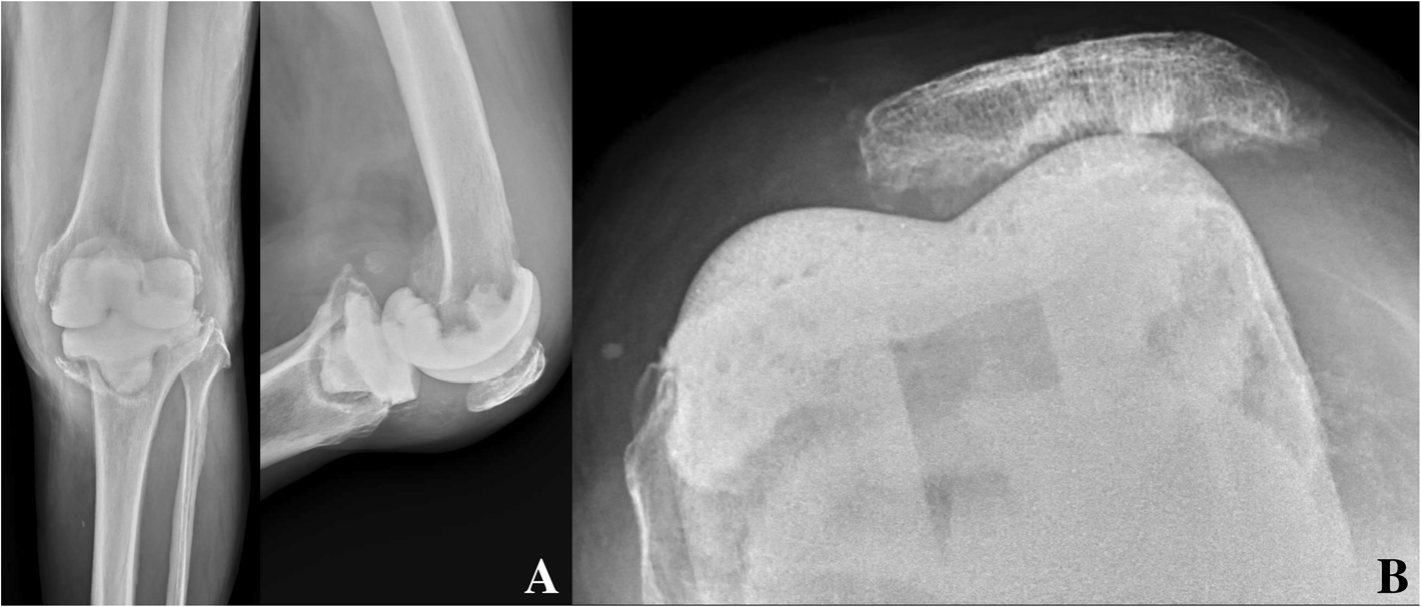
a Knee anteroposterior and lateral radiographs showing the medial plateau fracture with tibial spacer tilting 3 months after spacer insertion; b knee skyline Merchant radiograph showing patellar maltracking disorder 1 month after spacer insertion
